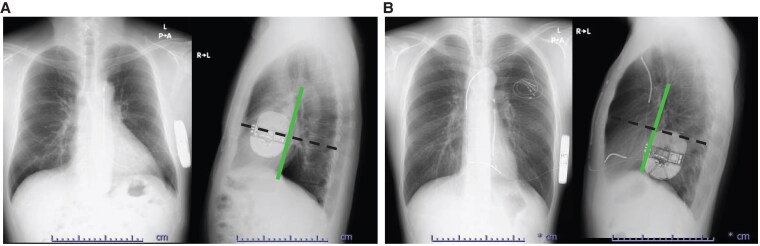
Chest X-rays of postero-anterior and lateral views after subcutaneous implantable cardioverter defibrillator implantation. The subcutaneous implantable cardioverter defibrillator generators are implanted anterior (*A*: Patient 1) and posterior (*B*: Patient 2) from the mid-lateral line (vertical line), respectively. According to the generator positions, the PRAETORIAN scores are different between Patients 1 (120) and 2 (60).
